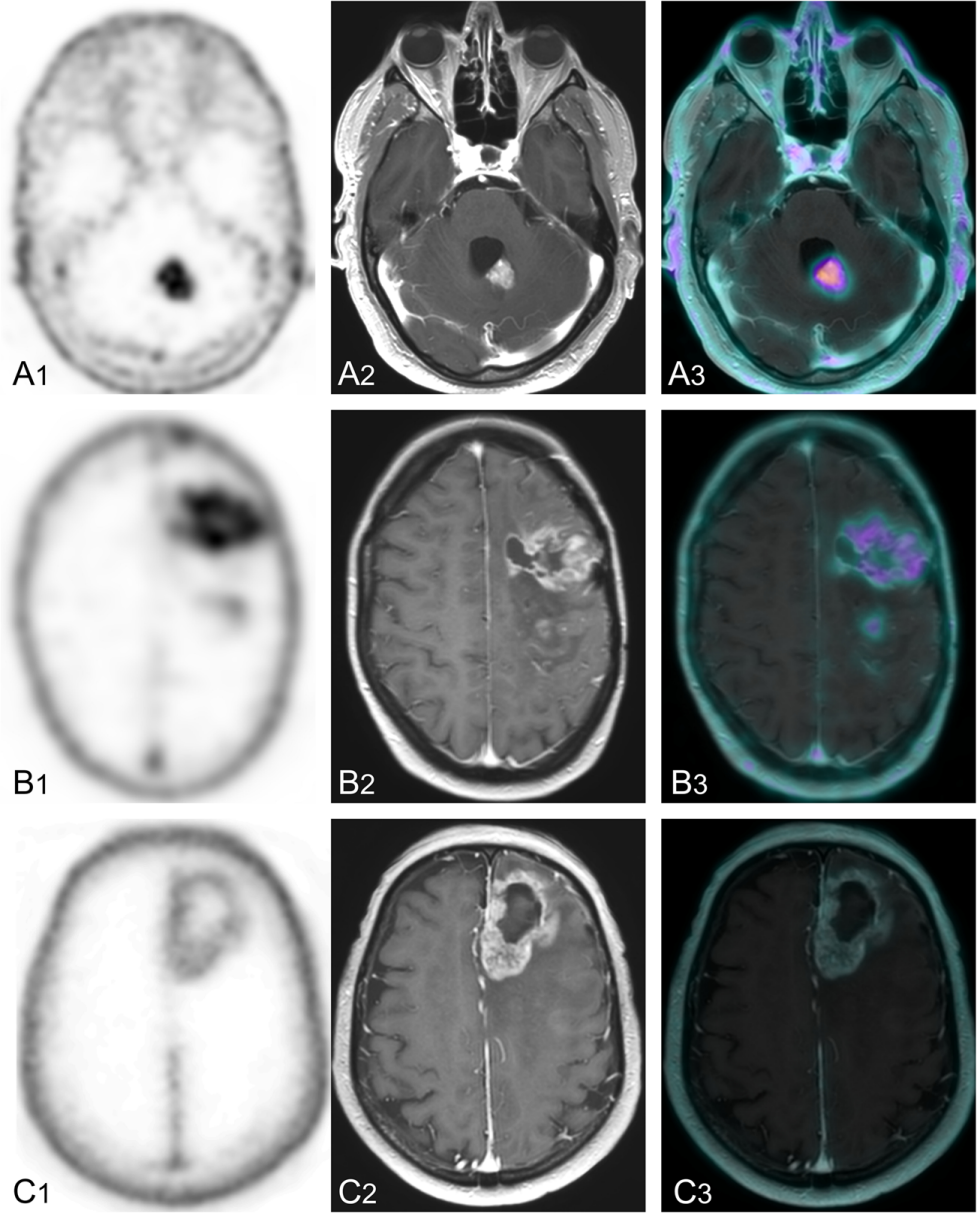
Axial [^68^Ga]Ga-Pentixafor PET (A1; B1; C1), T_2_-weighted MRI (A2; B2; C2) and fused [^68^Ga]Ga-Pentixafor PET/MRI (A3; B3; C3) images of three patients with suspicion of recurrent glioblastoma. (A) Male (patient no. 4 in Table 4) showing higher uptake (SUVmax 3.5) in the MR-enhancing tissue in the left cerebellar hemisphere compared to the other patients and than bloodpool activity (SUVmean 1.48). (B) Female (patient no. 2) showing low to moderate uptake (SUVmax 1.82) in the MR-enhancing tissue in the left frontal lobe slightly higher than bloodpool activity (SUVmean 1.23). (C) Male (patient no. 6) showing low uptake (SUVmax 1.46) in the MR-enhancing tissue in the left frontal lobe equal to bloodpool activity (SUVmean 1.48)
